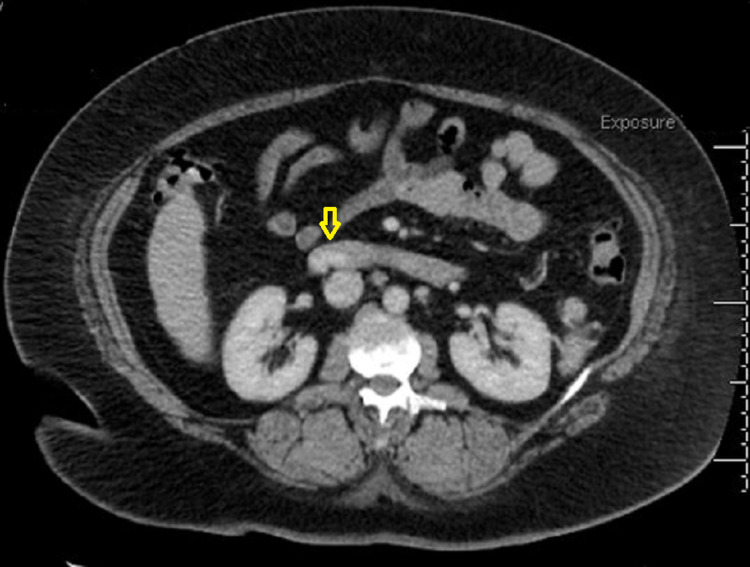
CT scan showing active bleeding in the proximal region of duodenum
